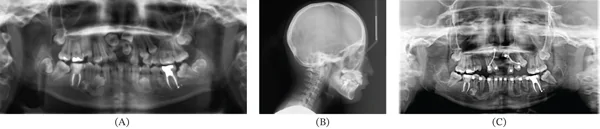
(A) Case #2 preliminary panoramic radiograph. (B) Case #2 preliminary lateral cephalogram. (C) Case #2 panoramic radiograph during treatment.
